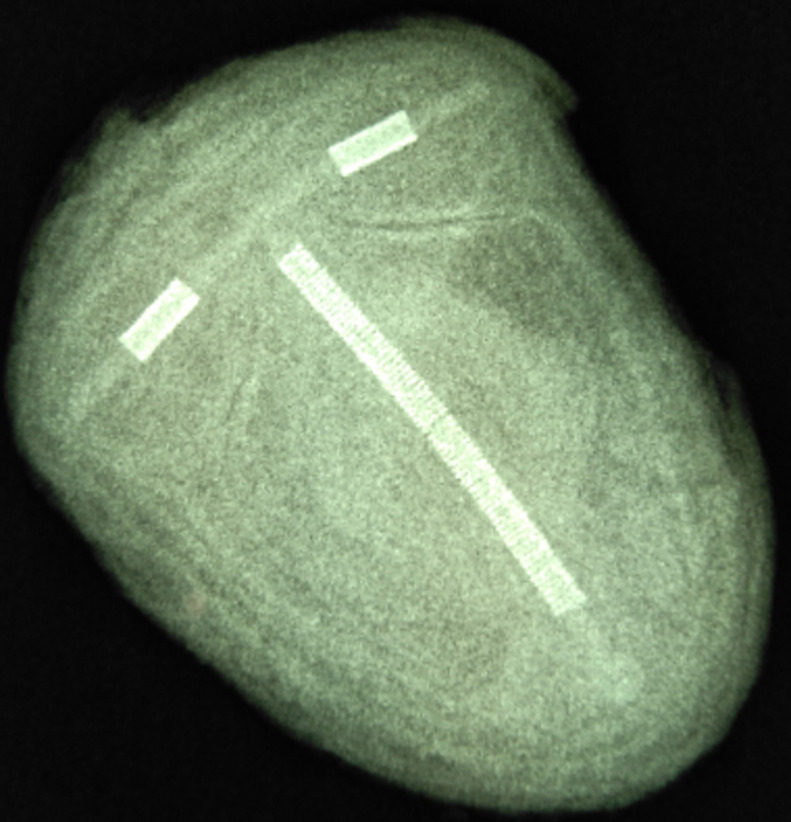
plain X-ray of the bladder stone; this reveals the intrauterine contraceptive device embedded in it
